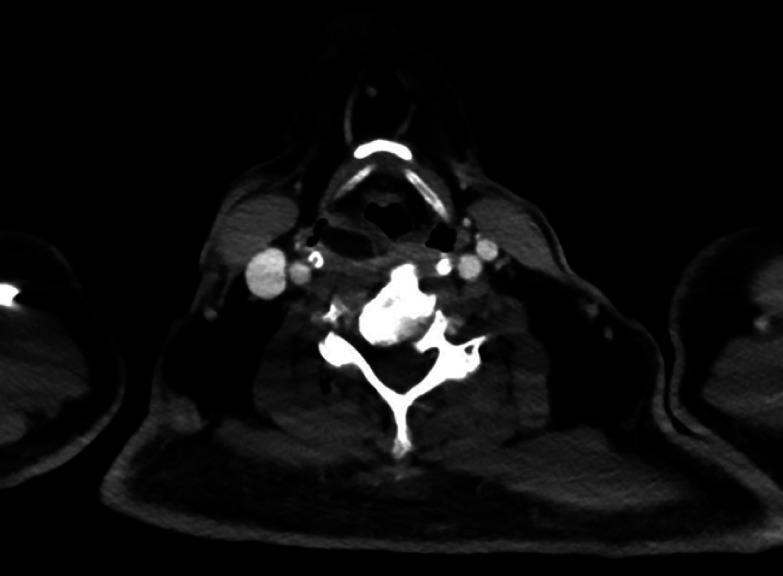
Computed tomography showing column-like region with a fat-like density in the larynx, without significant contrast enhancement.
